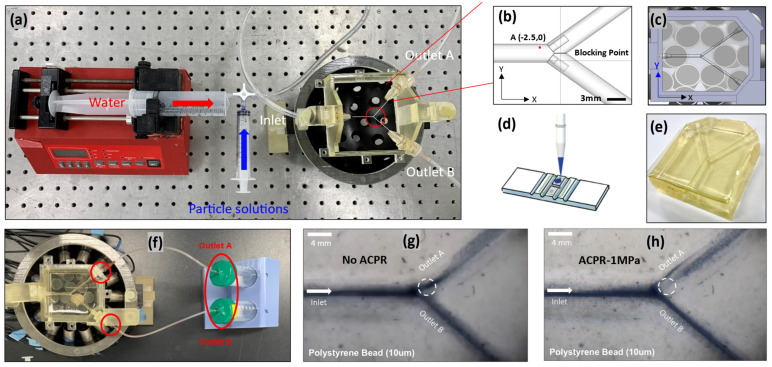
(a) Top experimental view of the fluid channel with specified inlet and outlets. (b,c) Animated description of the channel. (d) Hemocytometer counting chamber. (e) Gelatine-based bifurcation channel used in the experiment. (f) Fluid channel suspended on top of the ultrasonic transducers. (g,h) Images of particles flowing inside the fluid channel with and without external acoustic field, respectively.
